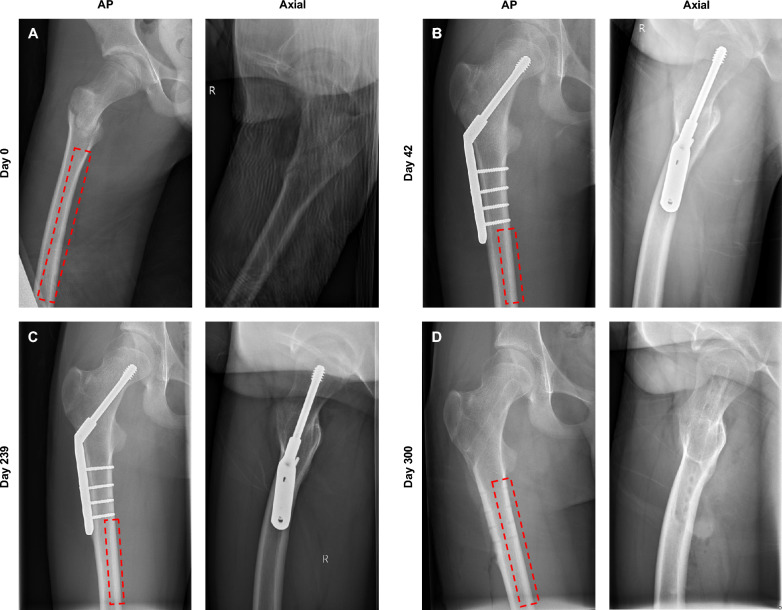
Radiographs of patient’s right hip in two planes (anteroposterior and axial) on day 0 (A), during the postoperative phase on day 42 (B) and on day 239 (C), and after implant removal on day 300 (D), with progressive increase in cortical thickness (red boxes)
